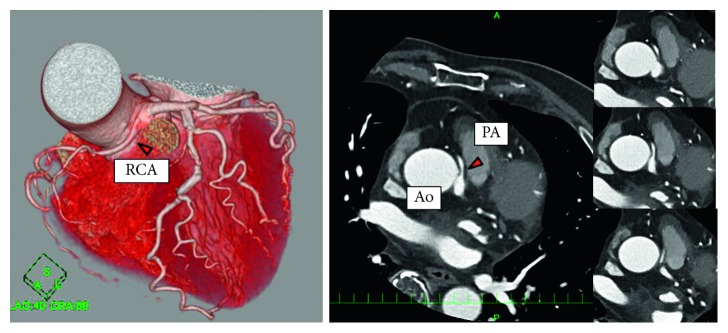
Coronary computed tomography revealing that the RCA originates from the left coronary sinus and passes between two great vessels. Ao = aorta, PA = pulmonary artery, and RCA = right coronary artery.
